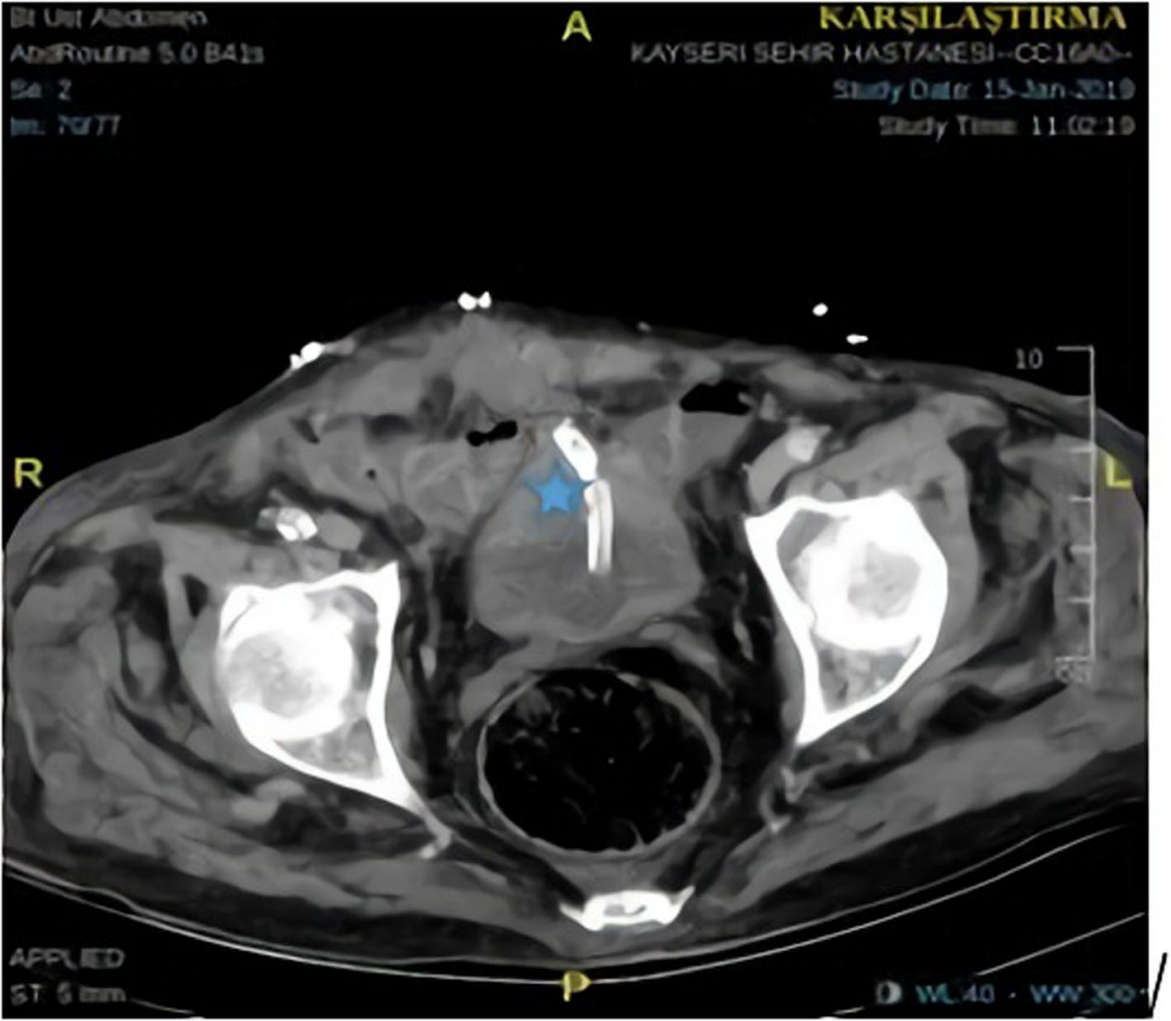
Pelvic CT axial image on the postoperative day 8 (*Foley catheter is seen in the bladder)
